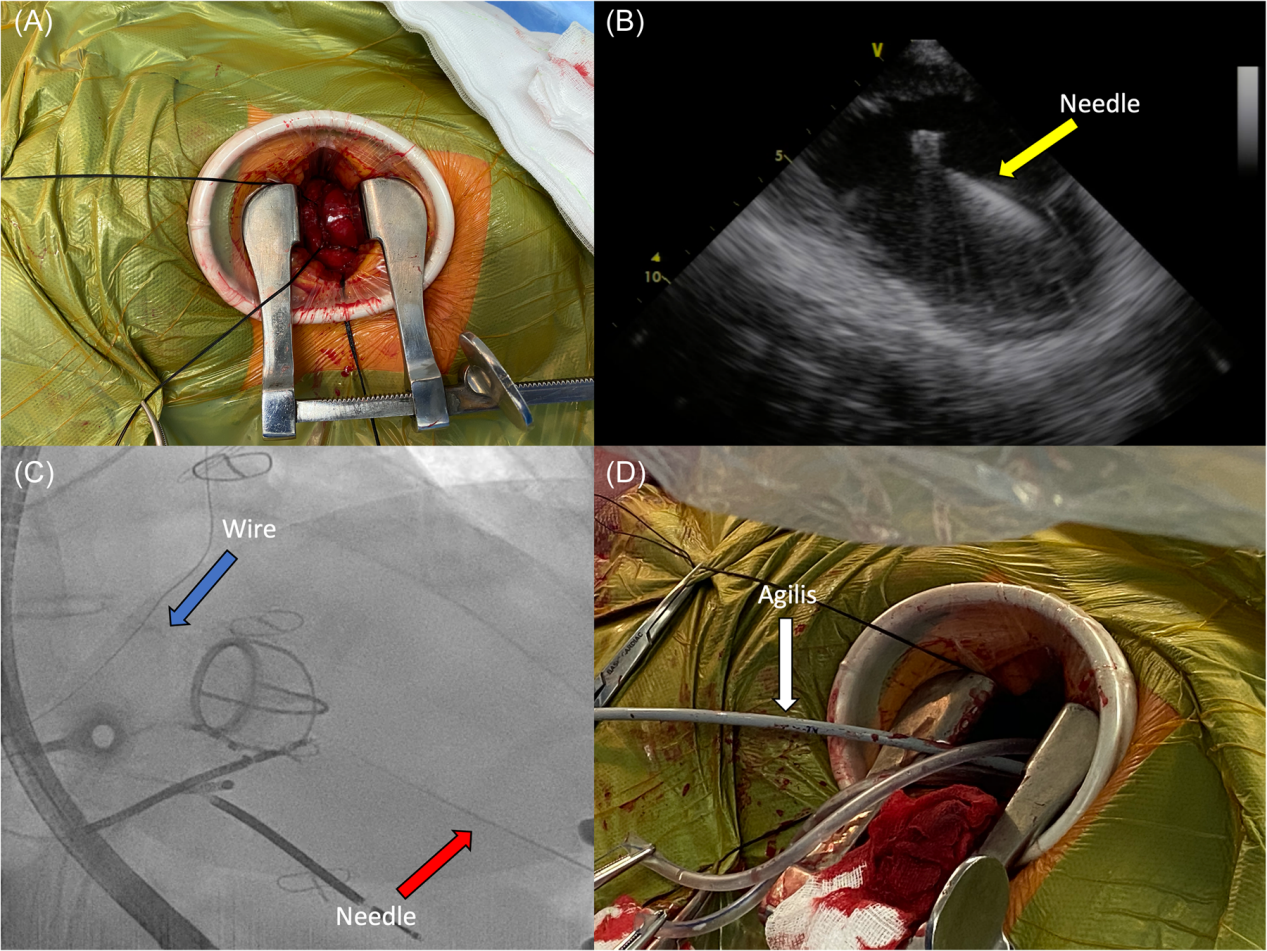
Surgical transapical access. (A) Mini‐thoracotomy revealing cardiac apex. (B) ICE image of apical puncture with a needle (white arrow). (C) Fluoroscopic image of apical puncture with a needle (red arrow) and guidewire advanced through the ball‐and‐cage mechanical valve into the left atrium (blue arrow). (D) Agilis EPI sheath in position through apical puncture (white arrow). ICE, intracardiac echocardiography
